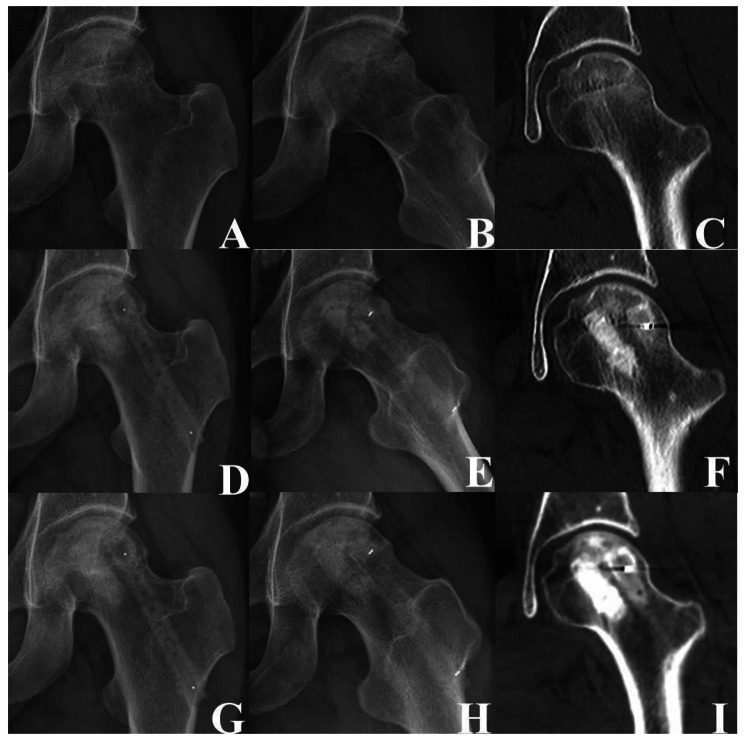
Images of a 36-year-old man who presented with type 1 left idiopathic osteonecrosis of the femoral head. (A, B) Preoperative anteroposterior and lateral radiographs showed that the femoral head was round. (C) Infarcts were present but did not involve the subchondral bone. (D–F) Three days after the operation, radiographs and computed tomography showed the shadow of the support rod and the bone graft. (G–I) Three years after the operation, radiographs and computed tomography showed that the femoral head was round and the bone density had increased
